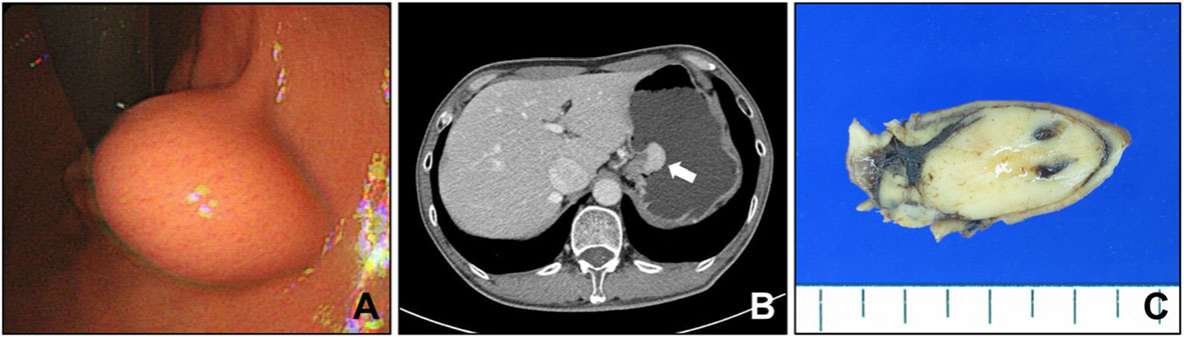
Endoscopic, radiologic, and gross features of the tumor. a Gastric submucosal mass protruded into the lumen and had an intact mucosa was observed *via* endoscopic examination. b Abdominal computed tomography shows a relatively well circumscribed polypoid mass with homogenous enhancement near the gastric cardia (arrow). c The cut surface of the tumor shows a relatively well circumscribed yellow-colored submucosal mass
